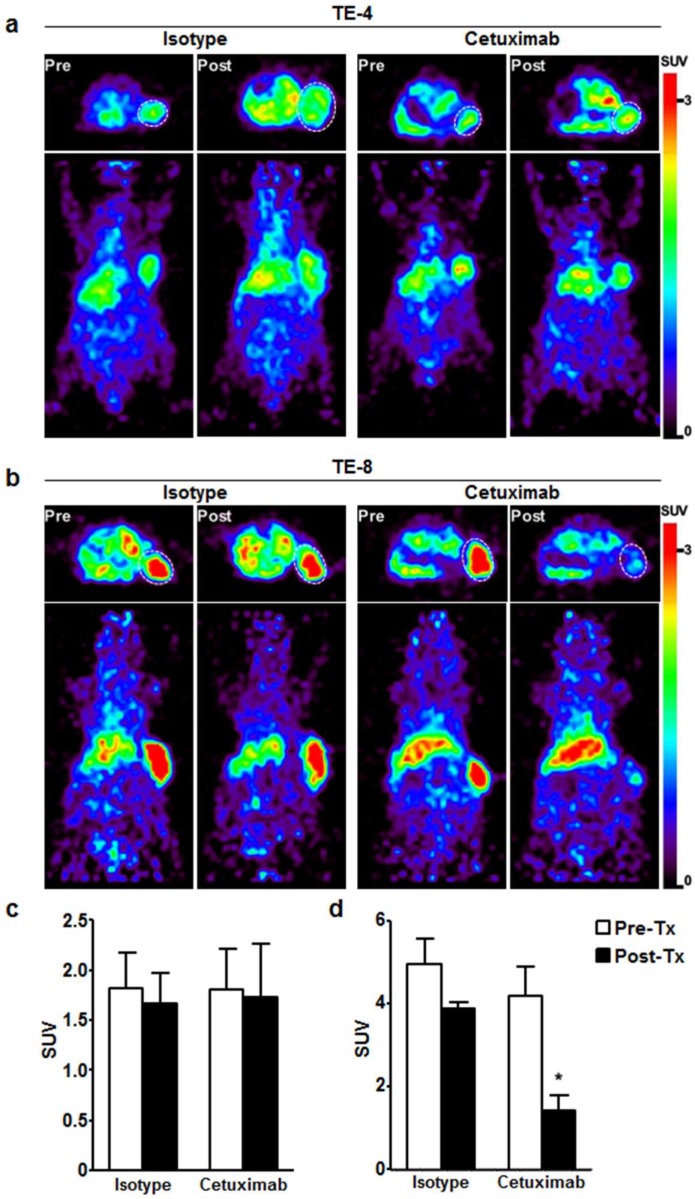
Immuno-PET imaging of EGFR expression level in ESCC xenograft model before and after cetuximab treatment. Transaxial (Top) and coronal (bottom) PET images of ^64^Cu-labeled cetuximab in (a) TE-4 and (b) TE-8 tumors determined EGFR level by cetuximab treatment. (a,c) ^64^Cu-labeled cetuximab uptake was not changed in TE-4 model with isotype or cetuximab treatment. (b,d) In TE-8 model, ^64^Cu-labeled cetuximab uptake was markedly reduced by only cetuximab treatment. (c) and (d) Quantitative indices of ^64^Cu-labeled cetuximab in TE-4 and TE-8 tumor represented as standardized uptake value (SUV). * *p* < 0.01. White dotted circle is tumor.
